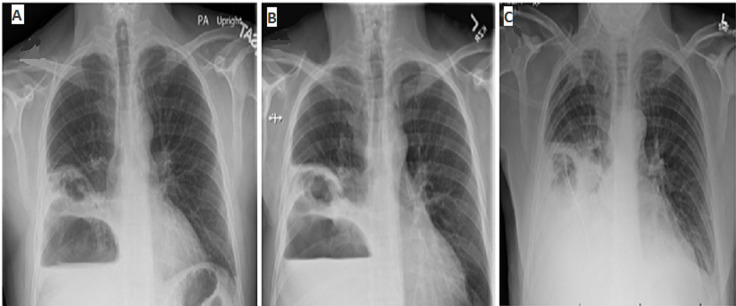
A) day 1 post-admission chest X-rays showing the development of large fluid-filled cavities; B) day 2 post-admission chest X-rays showing the development of large fluid-filled cavities; C) reduction in the airspace in the right hemithorax (day 3)
